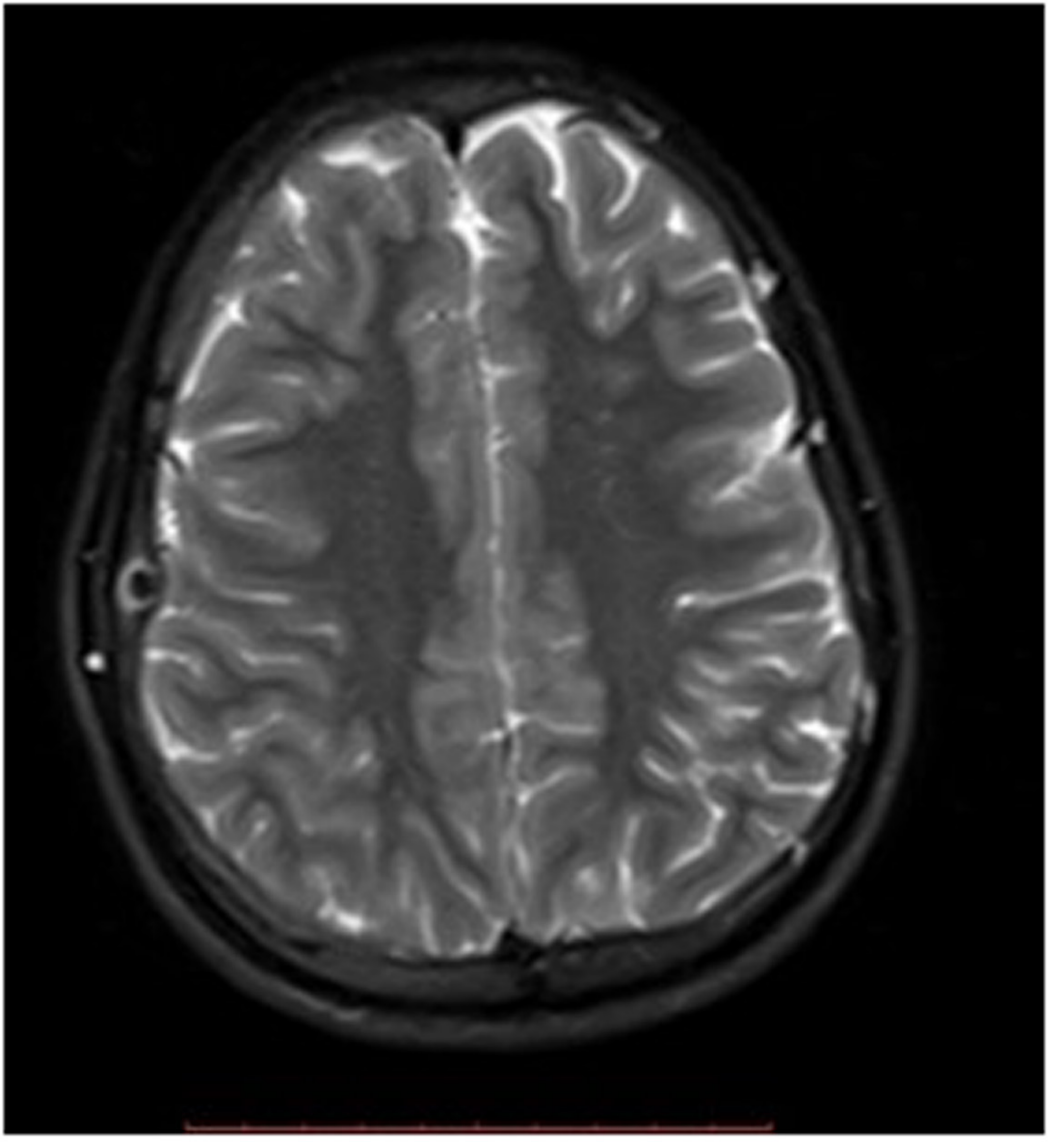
Axial T2‐weighted MR image shows a low signal intensity centrally with high signal intensity peripherally in the diploic space
